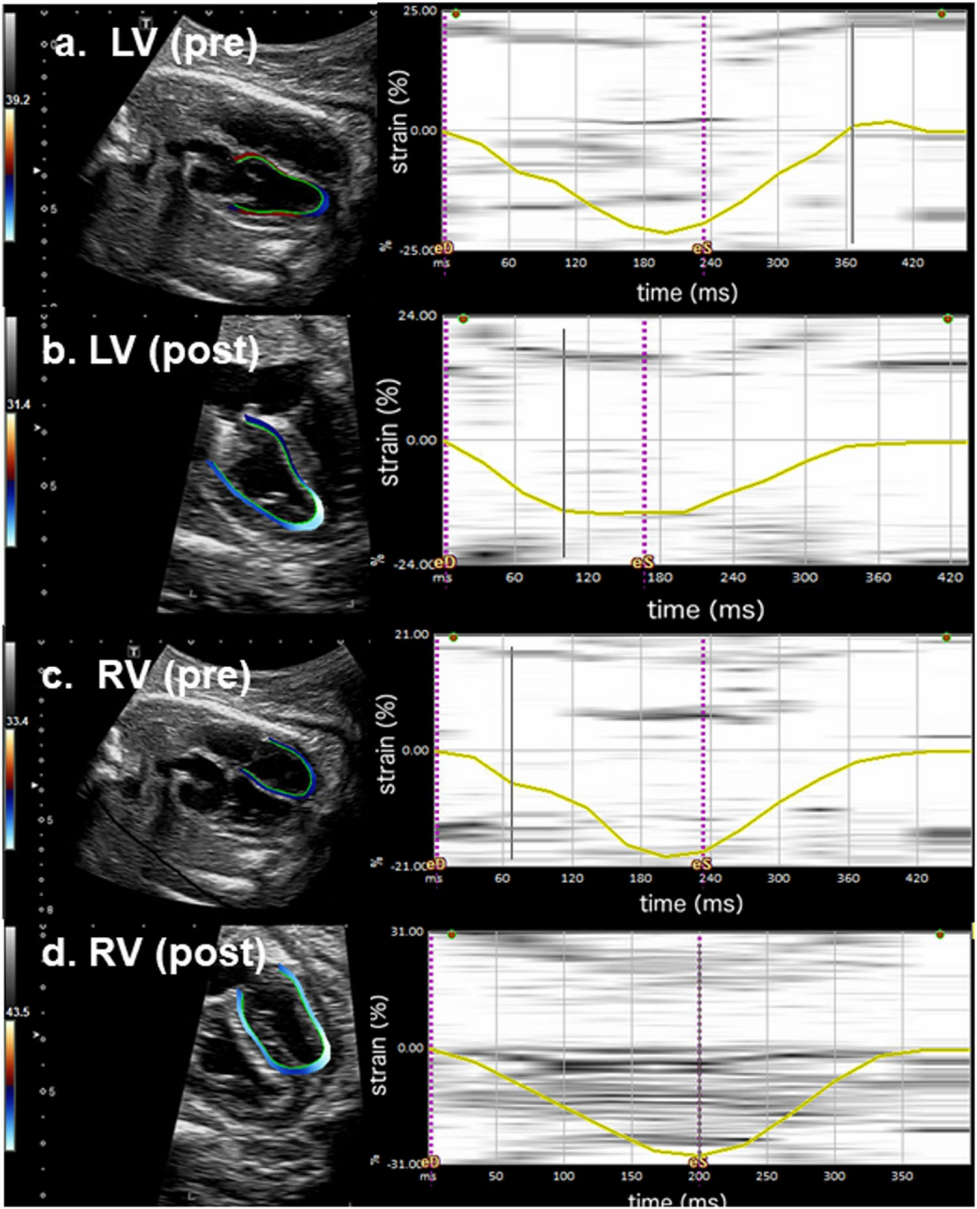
Speckle tracking echocardiography: Left panels-Speckle tracking echocardiography of the fetal lamb with 4-chamber-view and traced endocardial border of the ventricle. Right panels-Graphically displayed global longitudinal peak systolic strain (LPSS, yellow line) of one cardiac cycle; dotted purple line marking end-diastole (eD) and end-systole (eS). (a) Normal left-ventricular global myocardial deformation pattern pre-occlusion of the FO (LV pre) with global LPSS of −19.7%. (b) Post FO occlusion (LV post), the global LPSS is markedly reduced to −14.1%. (c) Normal right-ventricular global myocardial deformation pattern pre-occlusion of the FO (RV pre) with global LPSS of −18.6%. (d) Post FO occlusion (RV post), immediate increase of RV global LPSS to −29.3%.
